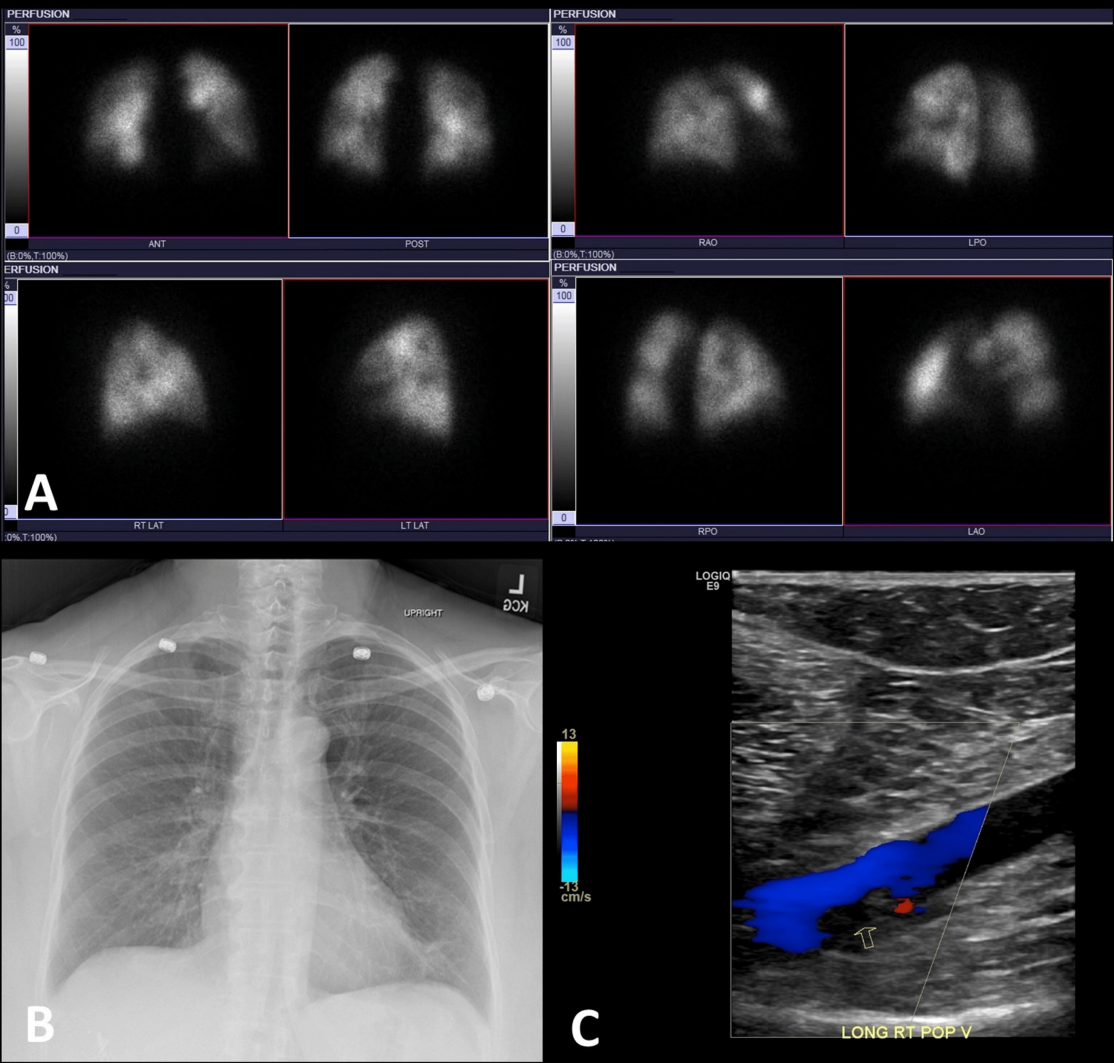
High probability for PE in a 66-year-old woman positive with COVID-19 (A) Images from lung perfusion scintigraphy performed with 99mTc-MAA, large-sized segmental perfusion defects in the posterior segment of the right upper lobe and inferior lingula. Moderate-sized segmental perfusion defects in the anteromedial basal segment of the left lower lobe and anterior basal segment of the right lower lobe. Based on modified PIOPED II perfusion-only criteria, the study is best classified as a high-probability scan for pulmonary embolism. (B) A chest radiograph is normal. (C) Color duplex study shows partially occlusive acute deep venous thrombosis within the right popliteal vein (yellow open arrow).
